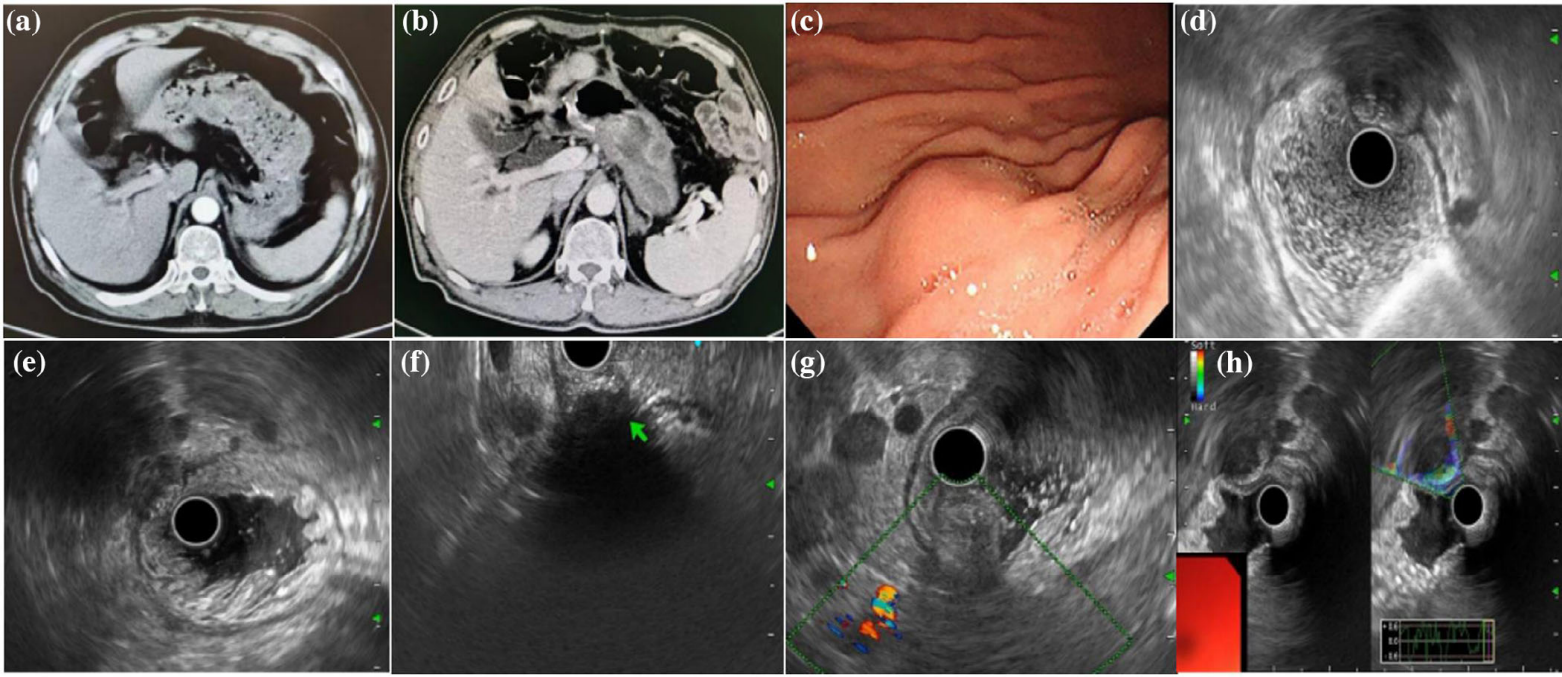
A case of gastric abscess from the Department of Gastroenterology, West China Hospital. (a) No nodule in enhanced computed tomography before surgery. (b) A newly emerged low‐density mass distant from the original surgical site in the submucosa of the gastric body. (c) Gastroscopy showed a hemispherical protuberant lesion appearing as a submucosal tumor. (d–f) 20 MHz probe, radial, and linear endoscopic ultrasound showed an oval, mixed hyperechoic and hypoechoic mass suspiciously originating from the submucosa or muscularis propria layer of the gastric wall. The endoscopic submucosal dissection boundary of mass was obscure and the serosa layer was unclear due to the influence of hyperechoic sound shadow. (g) There was no obvious blood flow inside the lesion under Doppler mode. (h) Mass was soft under Elastography mode. An area with nearly an echo and no signal for elastography indicated liquefaction inside.
